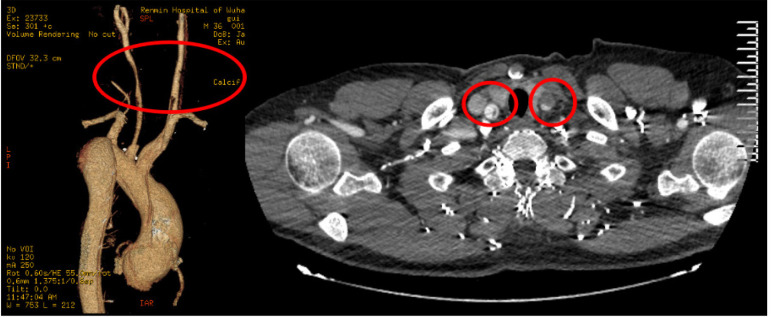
Computed tomography angiography of Case 1 suggesting a type A aortic dissection involving bilateral common carotid arteries (CCAs). The circle indicates a narrow true lumen in the left CCA and a large number of thromboses in the false lumen.
